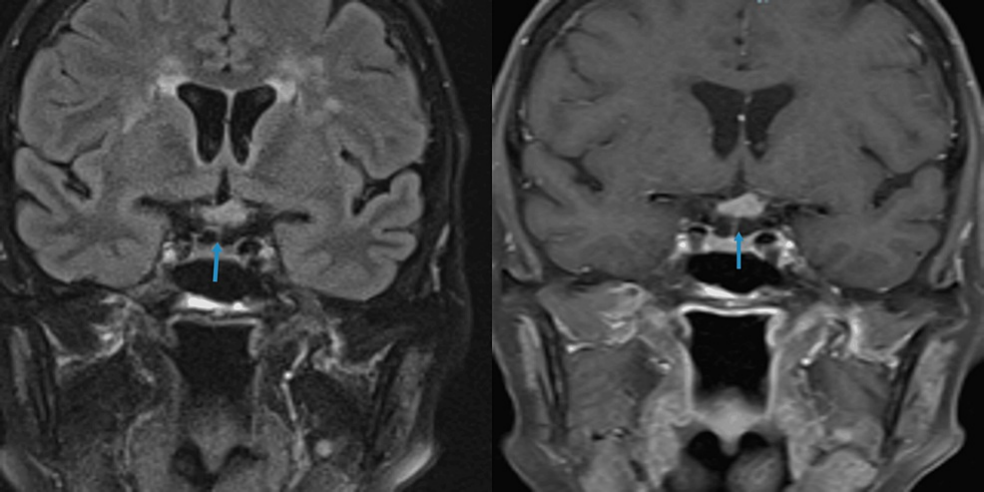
MRI orbits wwo contrast. T2 FLAIR (left) reveals signal hyperintensity and T1 with contrast in the coronal section (right) shows homogeneous enhancement of the left retro-orbital optic tract. wwo: With and without; FLAIR: Fluid-attenuated inversion recovery.
